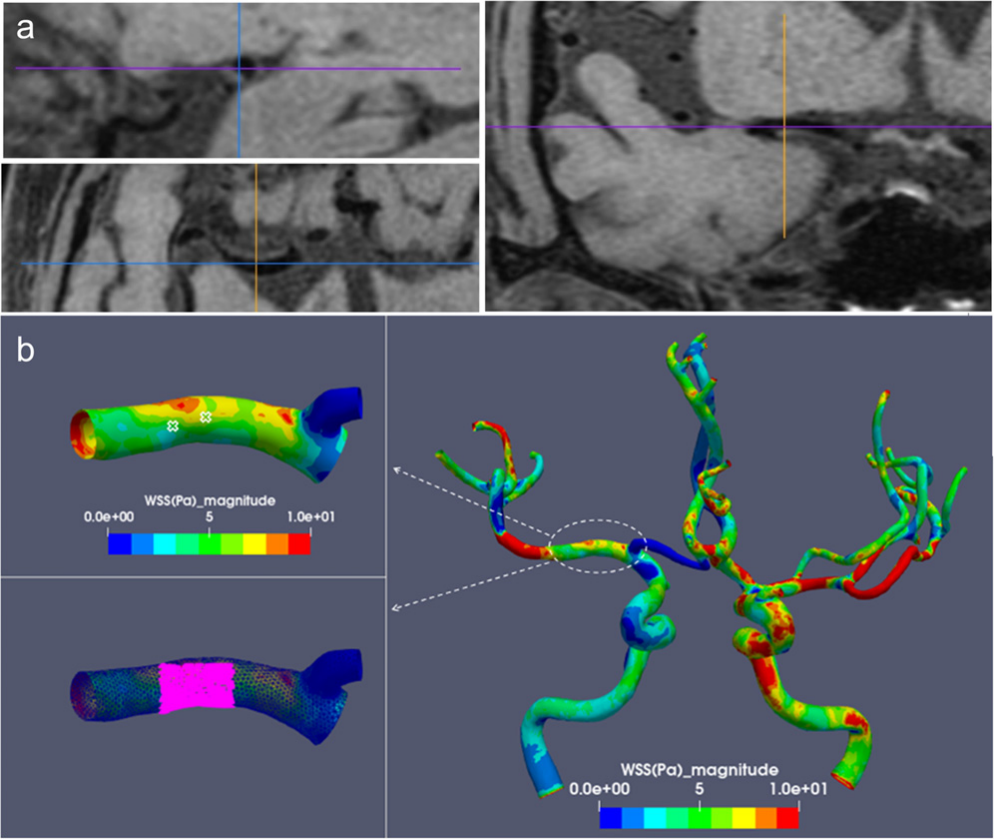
Plaque localization by a three-dimensional coordinate system and wall shear stress estimation on a three-dimensional reconstructed model. The plaque was selected on three-dimensional T1-weighted high-resolution magnetic resonance imaging (3D T1 HR-MRI). A coordinate (x, y, z) is shown based on a 3D coordinate system set preconditionally, which corresponds to the coordinate system of the 3D reconstructed wall shear stress (WSS) model. (a). The input coordinates (x, y, z) on 3D T1 HR-MRI are used to localize the selected point on the 3D reconstructed WSS model; each plaque is determined by 2-5 points; (b). The estimated WSS of the plaque-containing ventral and inferior walls; the cross sign indicates plaque location, and the pink spotted area represents the location of the WSS estimation.
